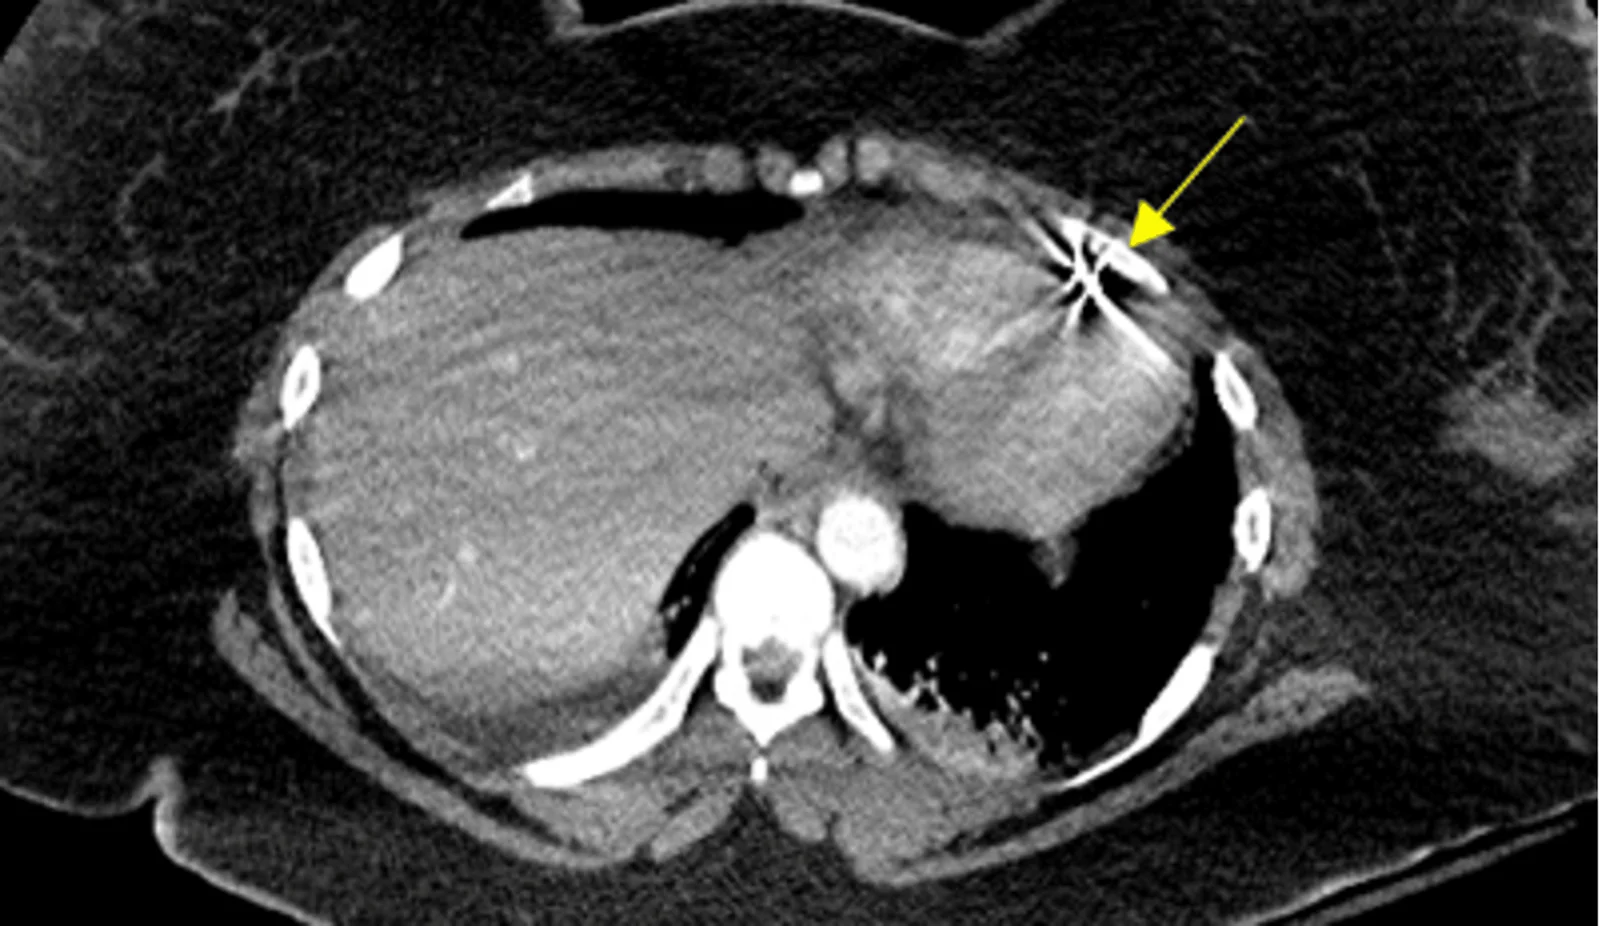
Representative CT image of a pellet at the apex of the heart.
